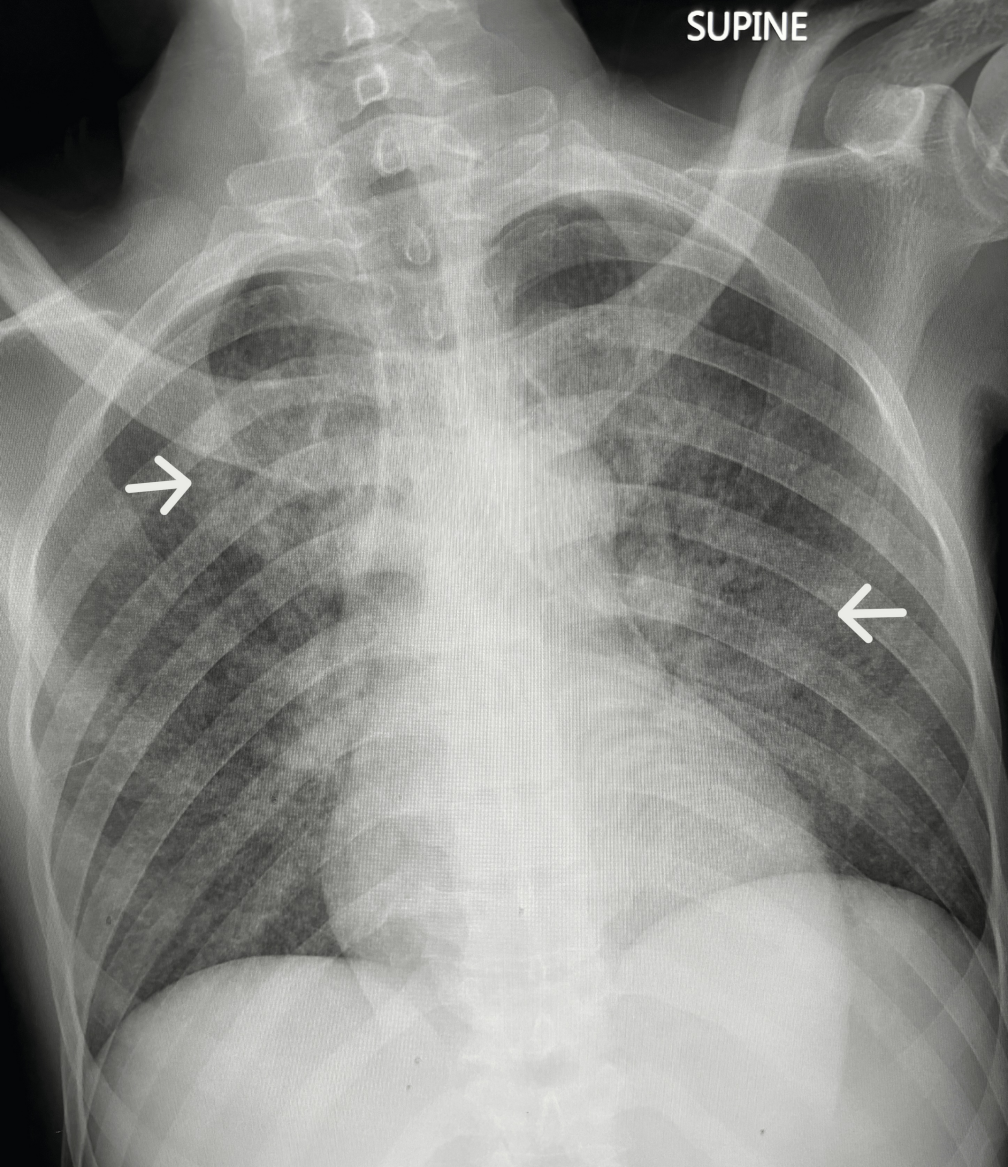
Anteroposterior chest roentgenogram demonstrating reticulonodular infiltrates (white arrows)
